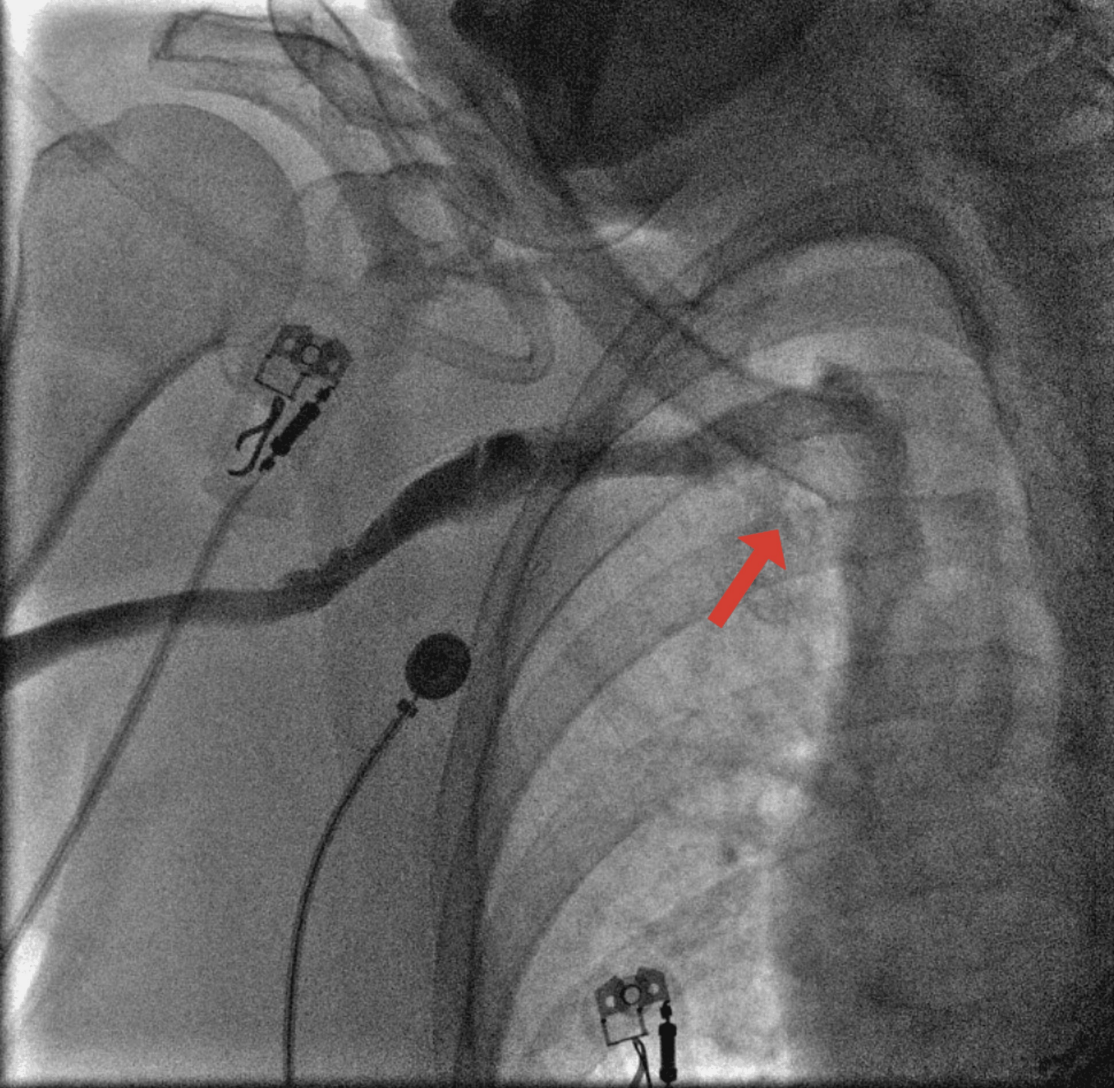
Chest X-ray angiography with contrast shows an acute angle of the subclavian vein on the right side.
